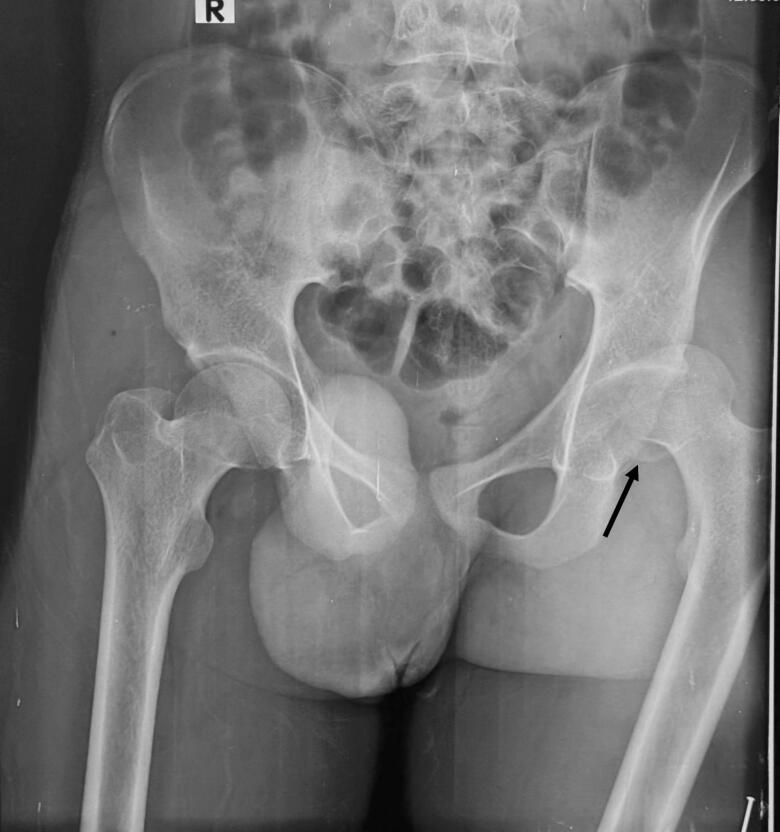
Pre-operative x-ray of pelvis with bilateral hip joint showing posterior dislocation of left hip with femoral head fracture confined to the inferior portion of the femoral head appearing as small, isolated fragment detached from the femoral head.
